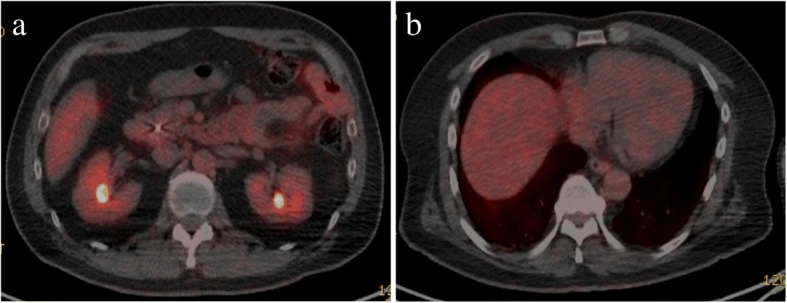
a Fluorodeoxyglucose positron emission tomography (FDG-PET) after chemotherapy showed no uptake in the tumor in the duodenum or the para-aortic lymph nodes. b FDG-PET after chemotherapy showed no uptake in segment 8 of the liver
